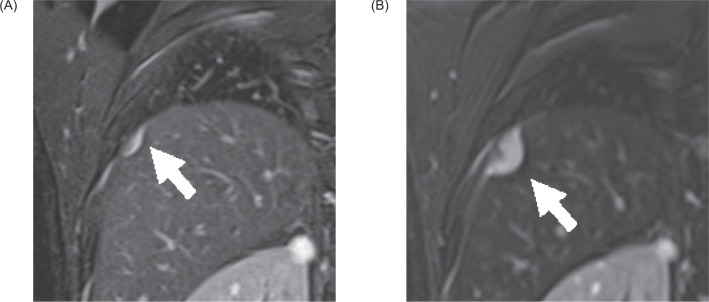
Plain MRI (fat-suppression T2WI). (A) Coronal MRI 6 months before resection revealed a 1.5-cm extrahepatic nodule. (B) Coronal MRI 2 months before resection revealed that the nodule had increased to 2.5 cm in diameter. The nodule was contiguous with the diaphragm and was compressing the liver from outside.
